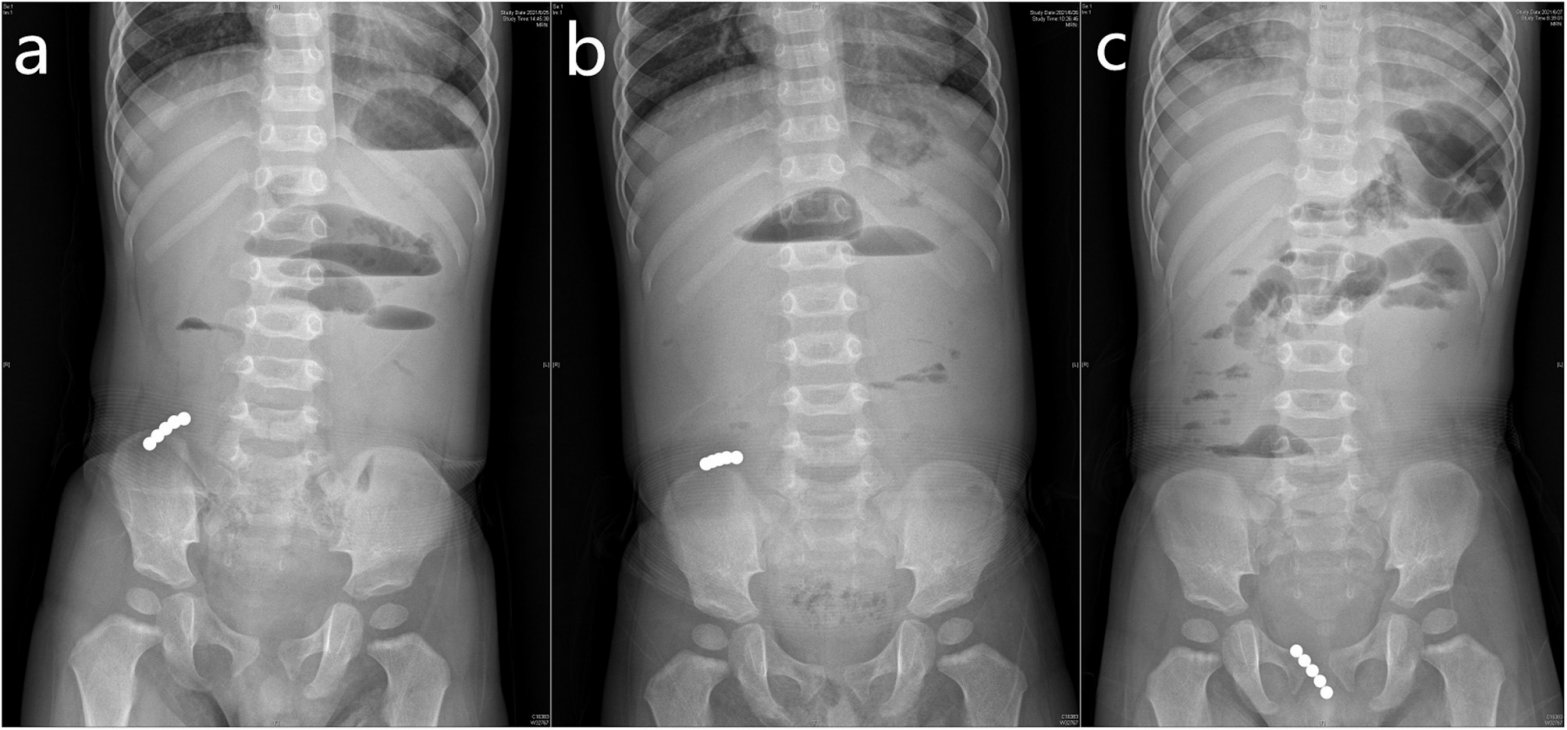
Plain abdominal and pelvic radiographic imaging revealed the progression of the Buckyballs. The Buckyballs passed spontaneously on 5 post-ingestion days and partial small bowel obstruction relieved.
